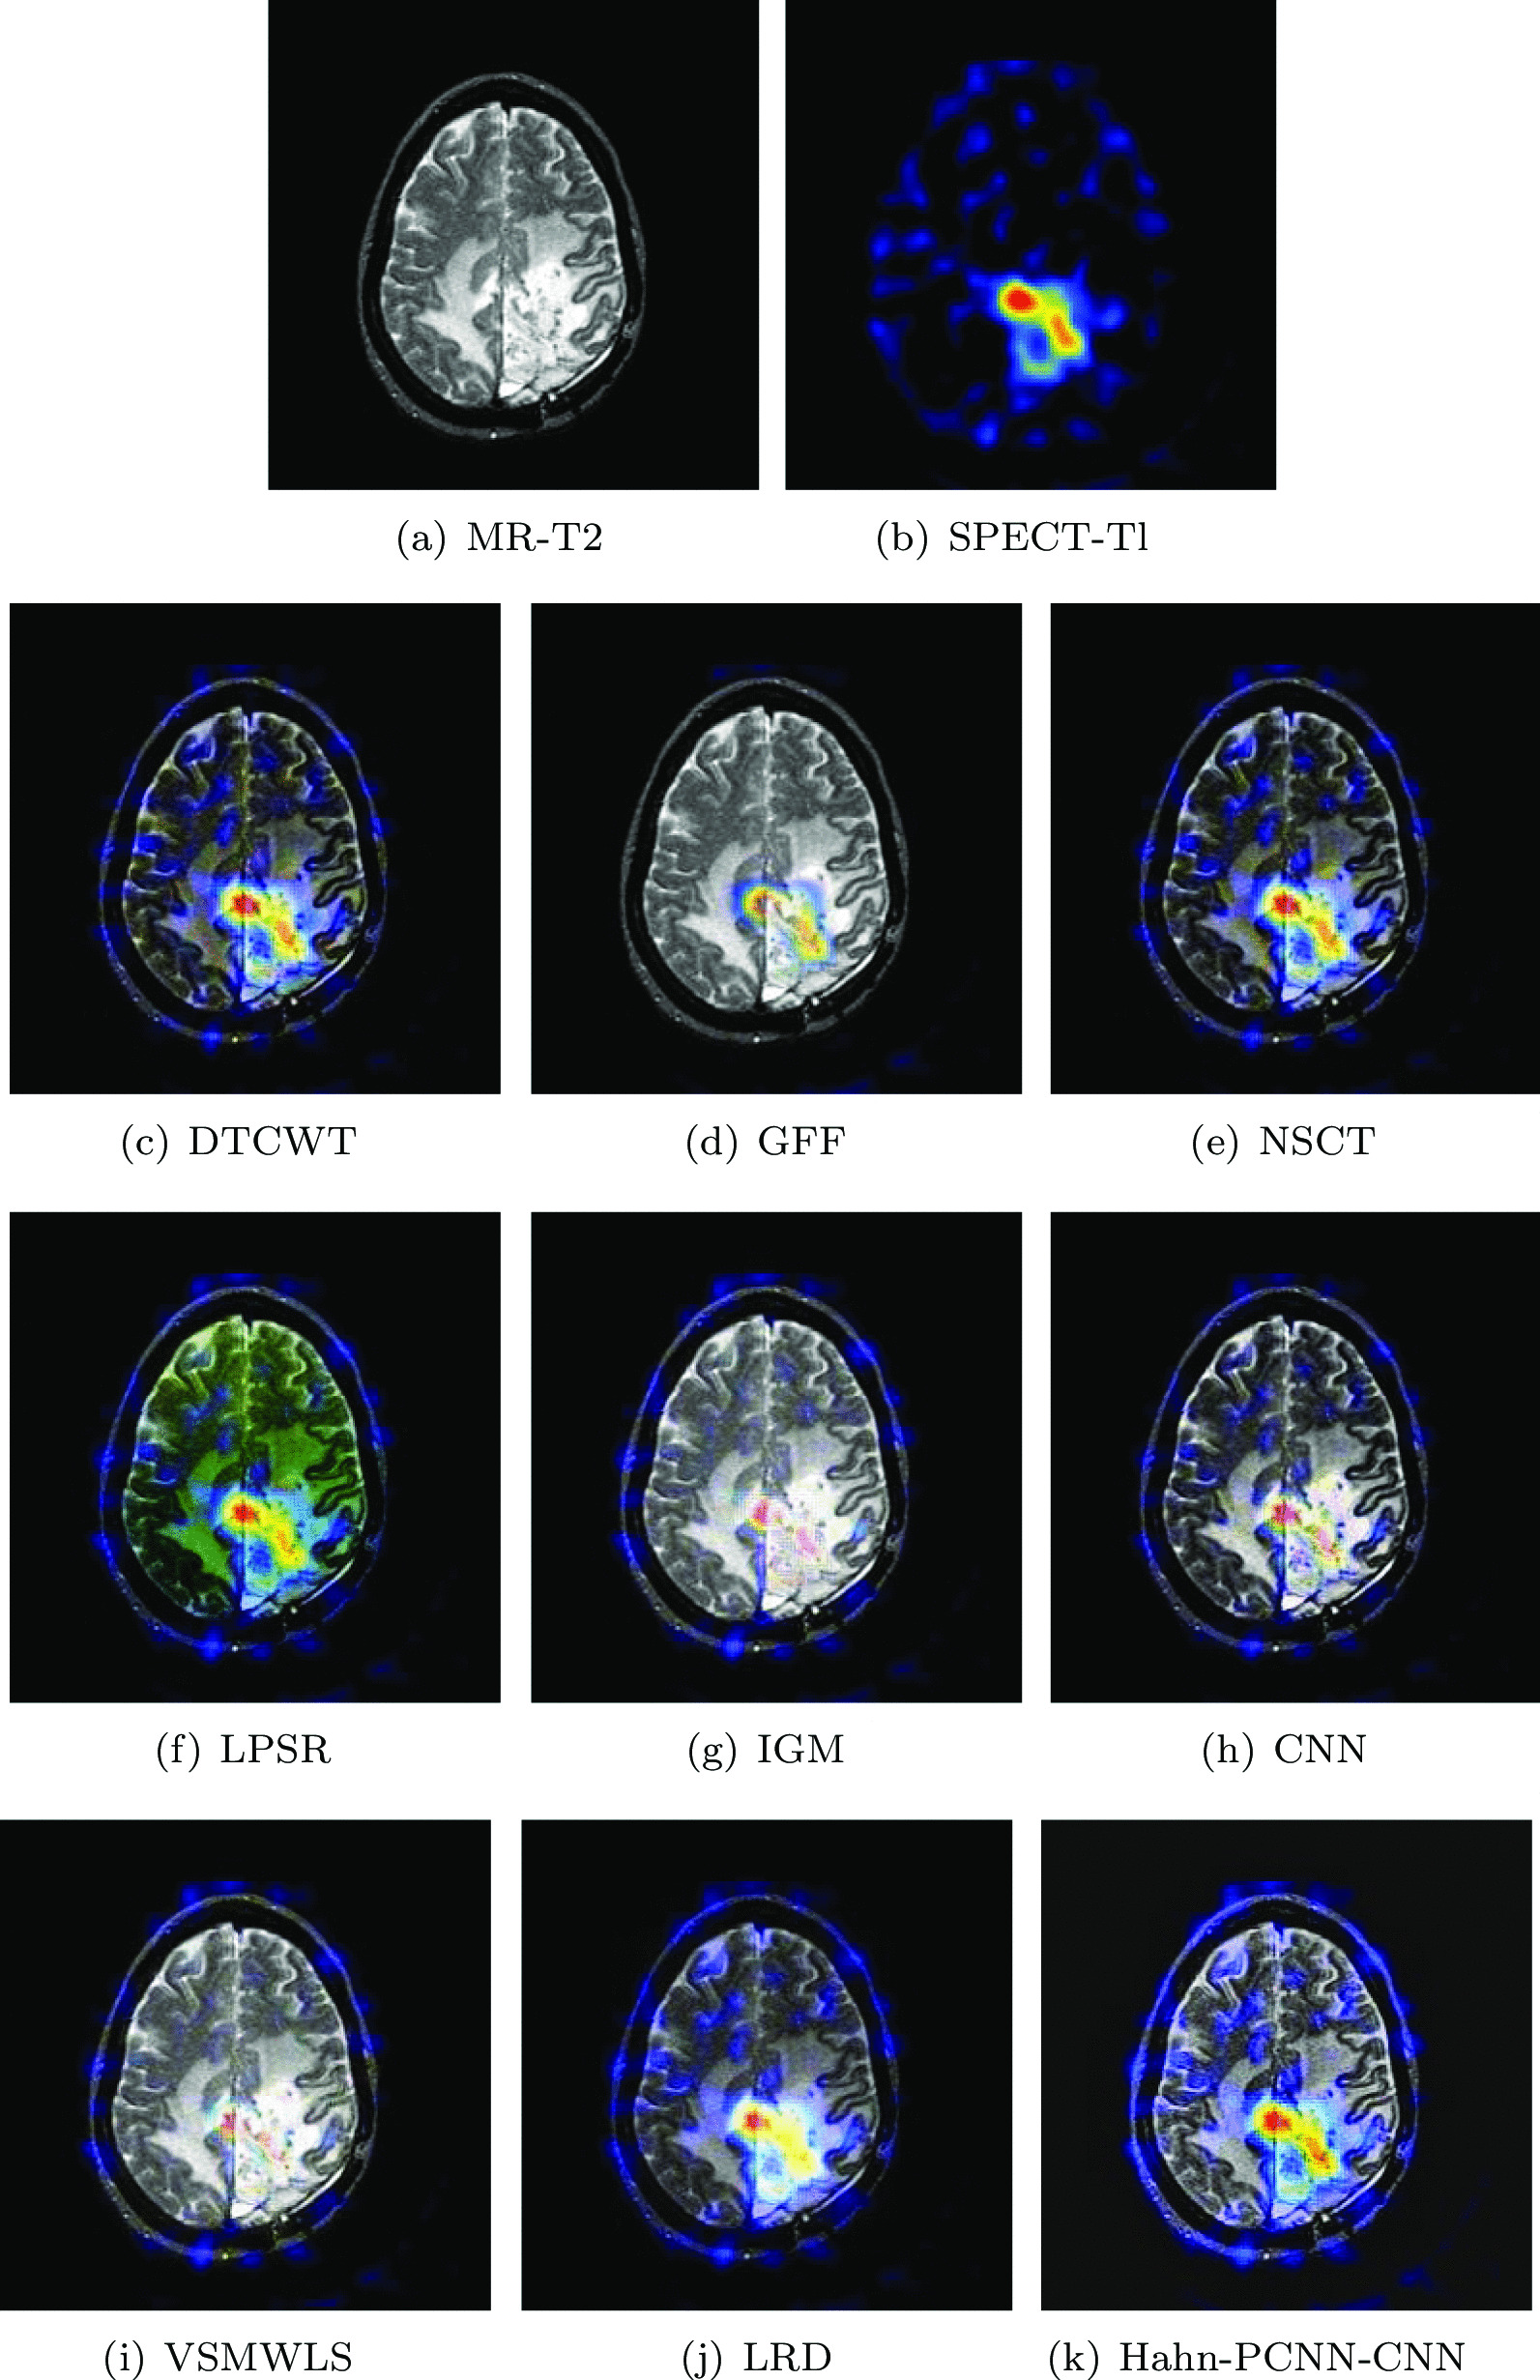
The first set of fused MRI-SPECT images from 9 methods on glioma
